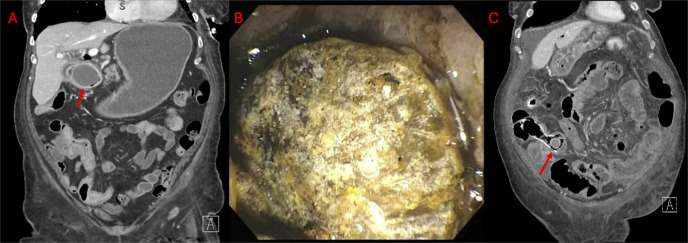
(A) Coronal CT abdomen and pelvis demonstrating gallstone within the duodenal bulb and resulting gastric distention. (B) Gallstone located at the duodenal bulb visualized on EGD. (C) Coronal CT abdomen and pelvis demonstrating gallstone fragment within the small bowel leading to small bowel obstruction.
